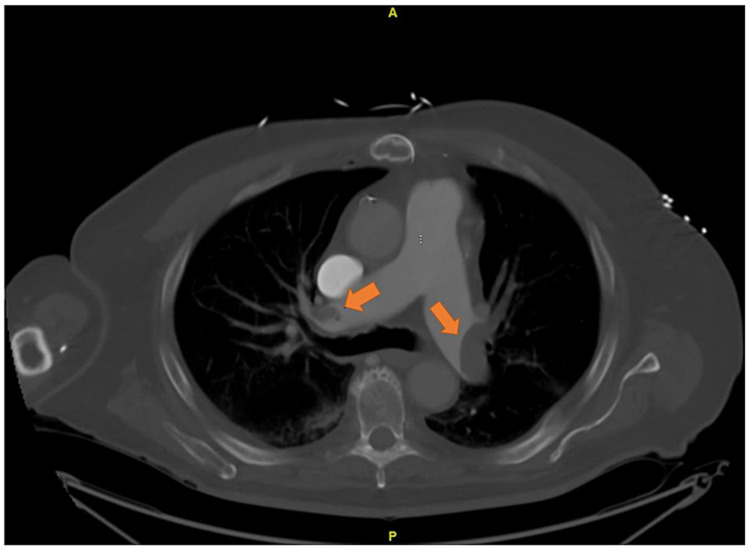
CT of the chest showing large bilateral clot burden (arrows) CT: computed tomography
